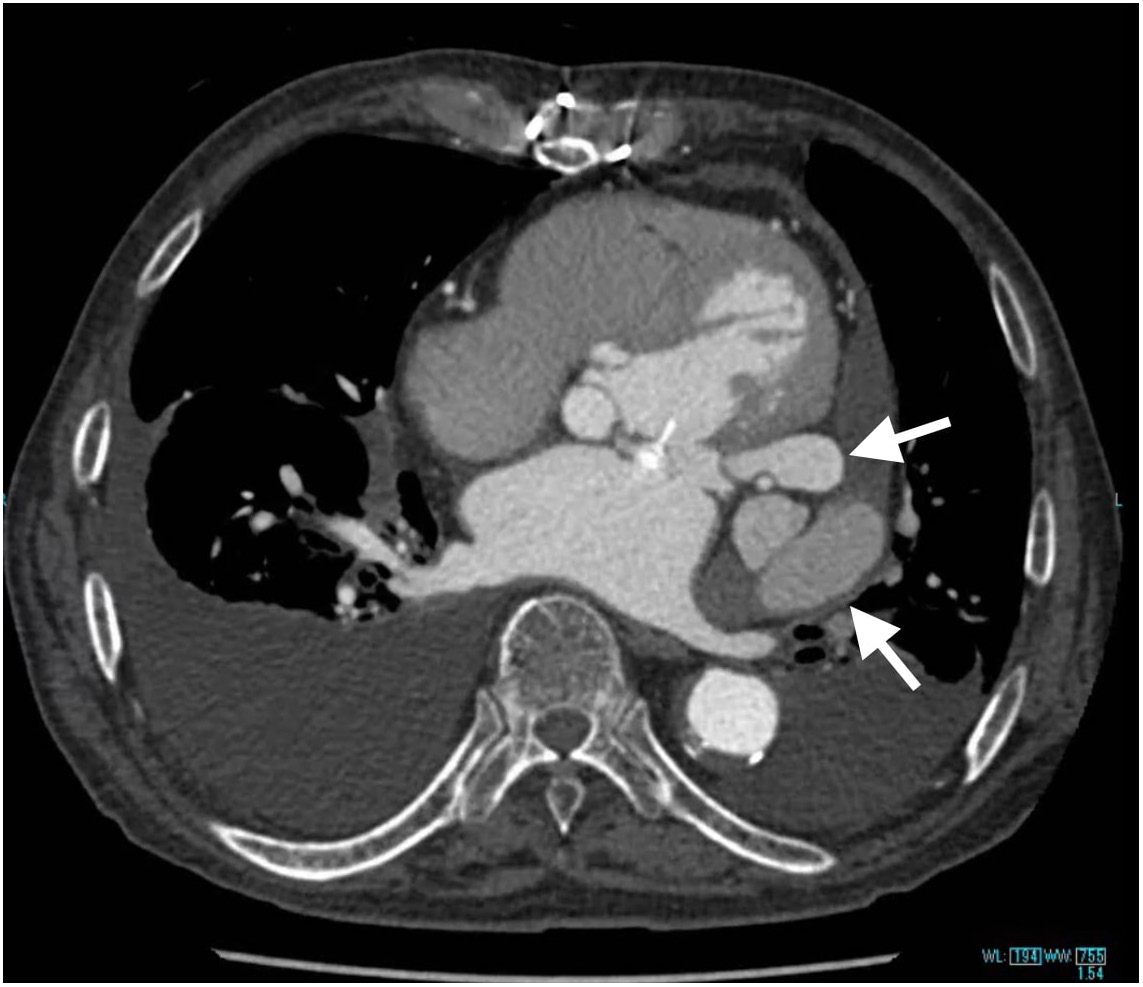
Coronal view of computed tomography imaging showing the pseudoaneurysm at the posterior wall of the left ventricular (arrow)
